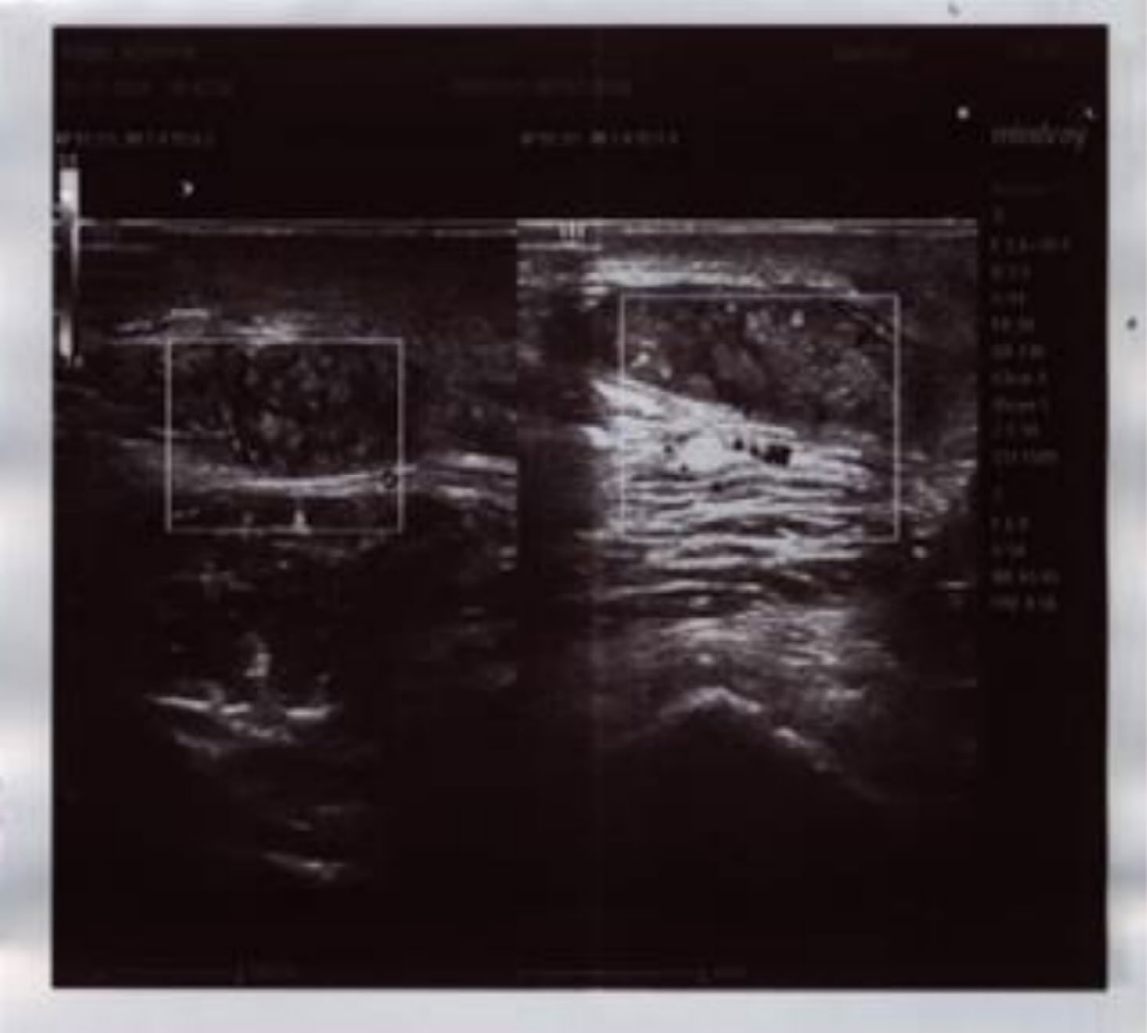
Ultrasound Scan of the lesion showing grains within pockets.
